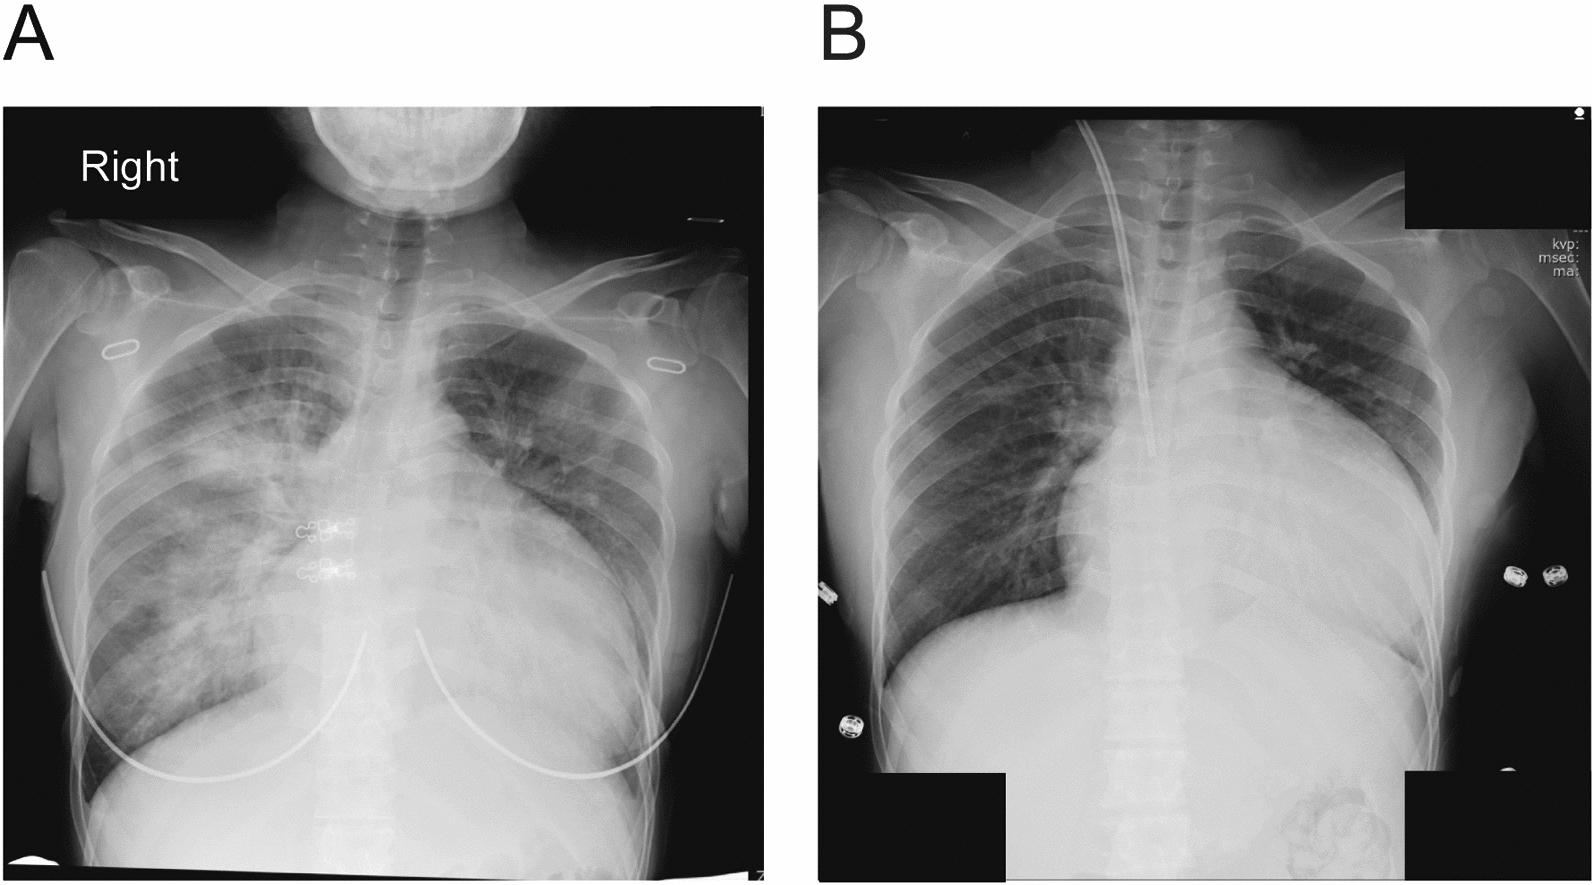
X-ray photographs before and after cesarean section. A X-ray at 22 weeks of gestation shows cardiac enlargement and decreased lung field permeability. B X-ray immediately after cesarean section shows no change in cardiac enlargement but improved lung field permeability
